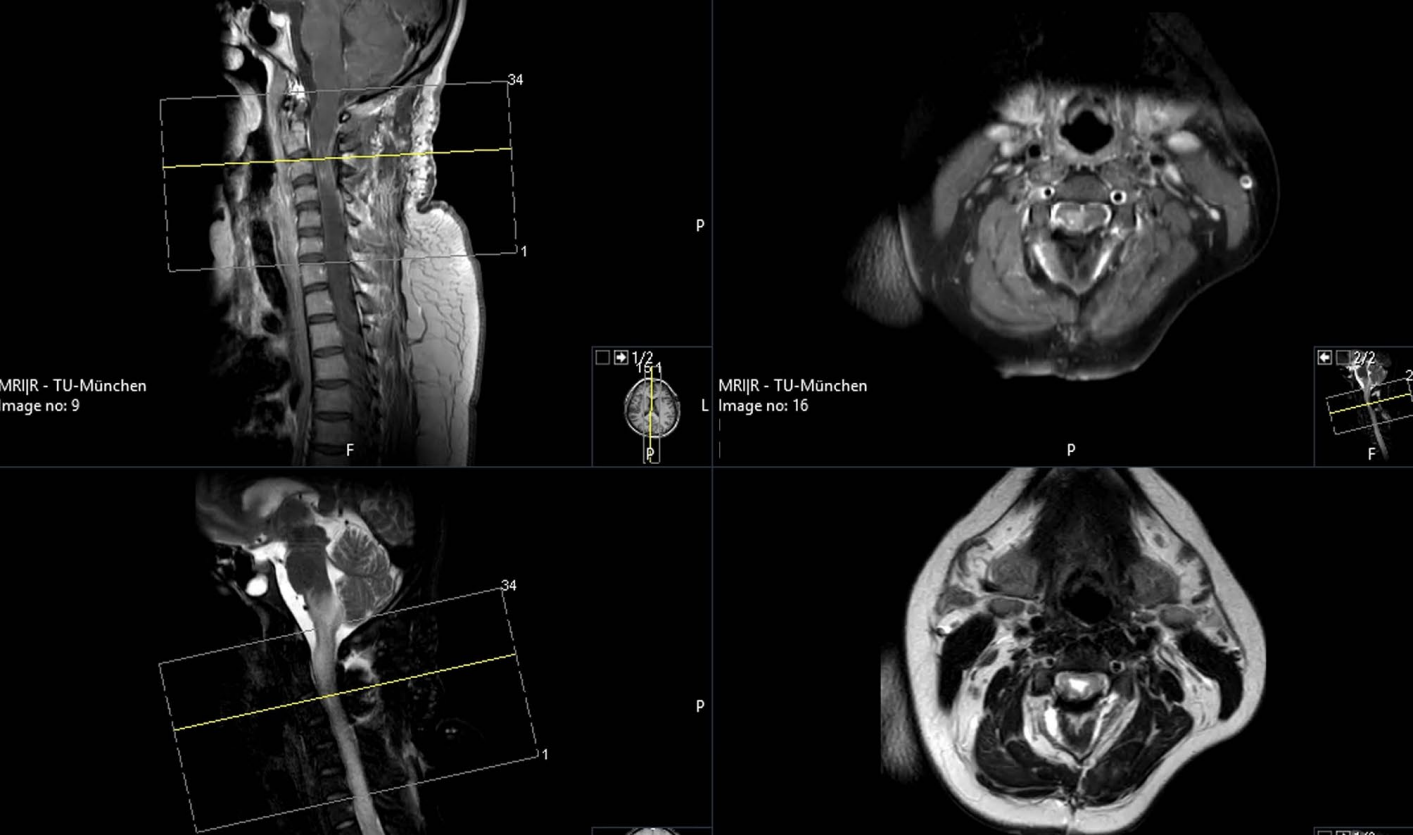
T1-MRI scan with contrast enhancement (upper image) and T2-MRI scan (lower image) confirming complete resection of the lesion
